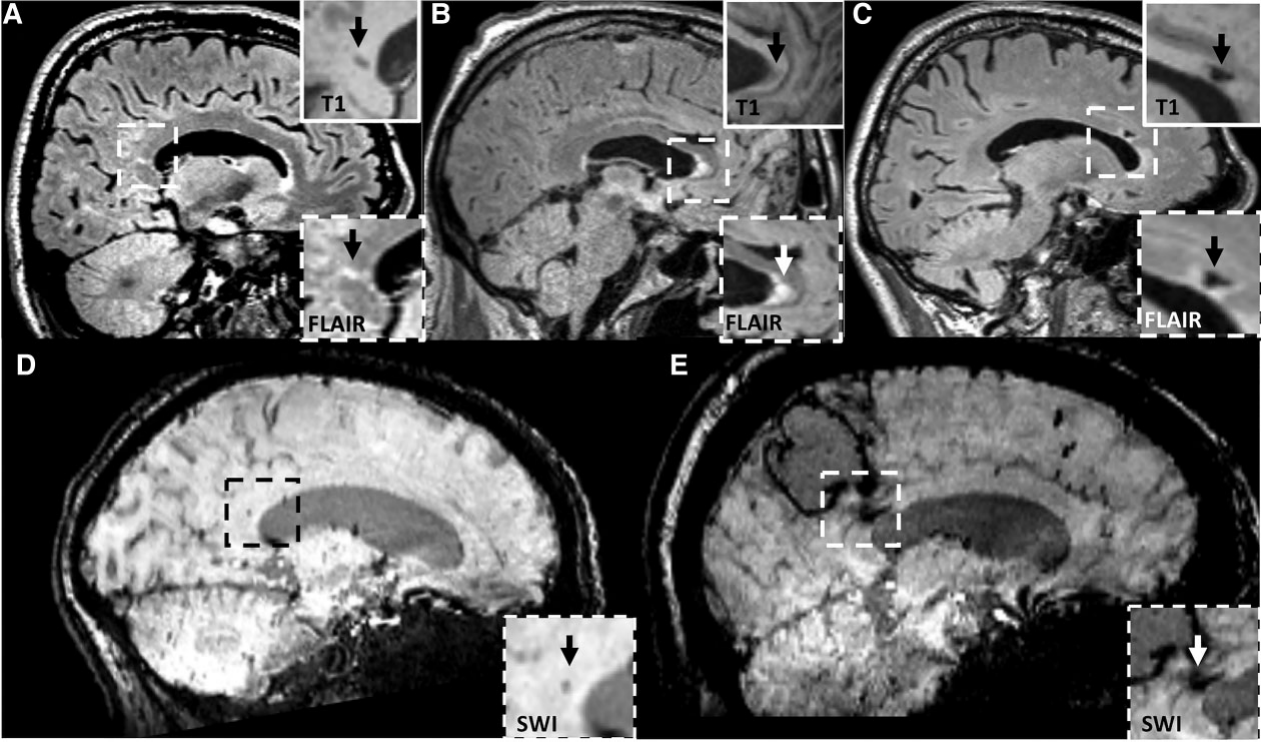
Representative examples of CC lesions in patients with CAA. (A) Cerebral microinfarct located in the splenium of the corpus callosum. (B) Diffuse FLAIR hyperintensity located in the genu of the corpus callosum. (C) Lacunar infarct located in the genu of the corpus callosum. (D) Cerebral microbleed located in the splenium of the corpus callosum. (E) Extension of intracerebral haemorrhage into the splenium of the corpus callosum.
